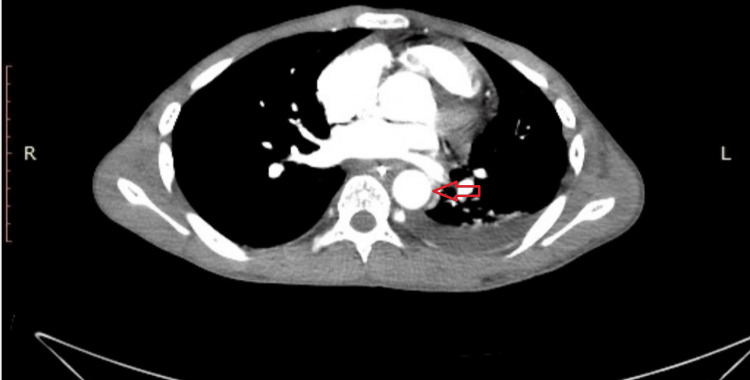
Postoperative computed tomography angiography (CTA) image Axial CTA image of the chest demonstrates the successful exclusion of the traumatic aortic dissection. The image shows a patent graft in the descending thoracic aorta with no evidence of a graft leak, pseudoaneurysm, or other graft-related complications. The aortic contour is regular, and there is complete resolution of the false lumen (arrow). A residual, non-expanding left-sided pleural effusion is also noted, which is a common finding following thoracotomy.
